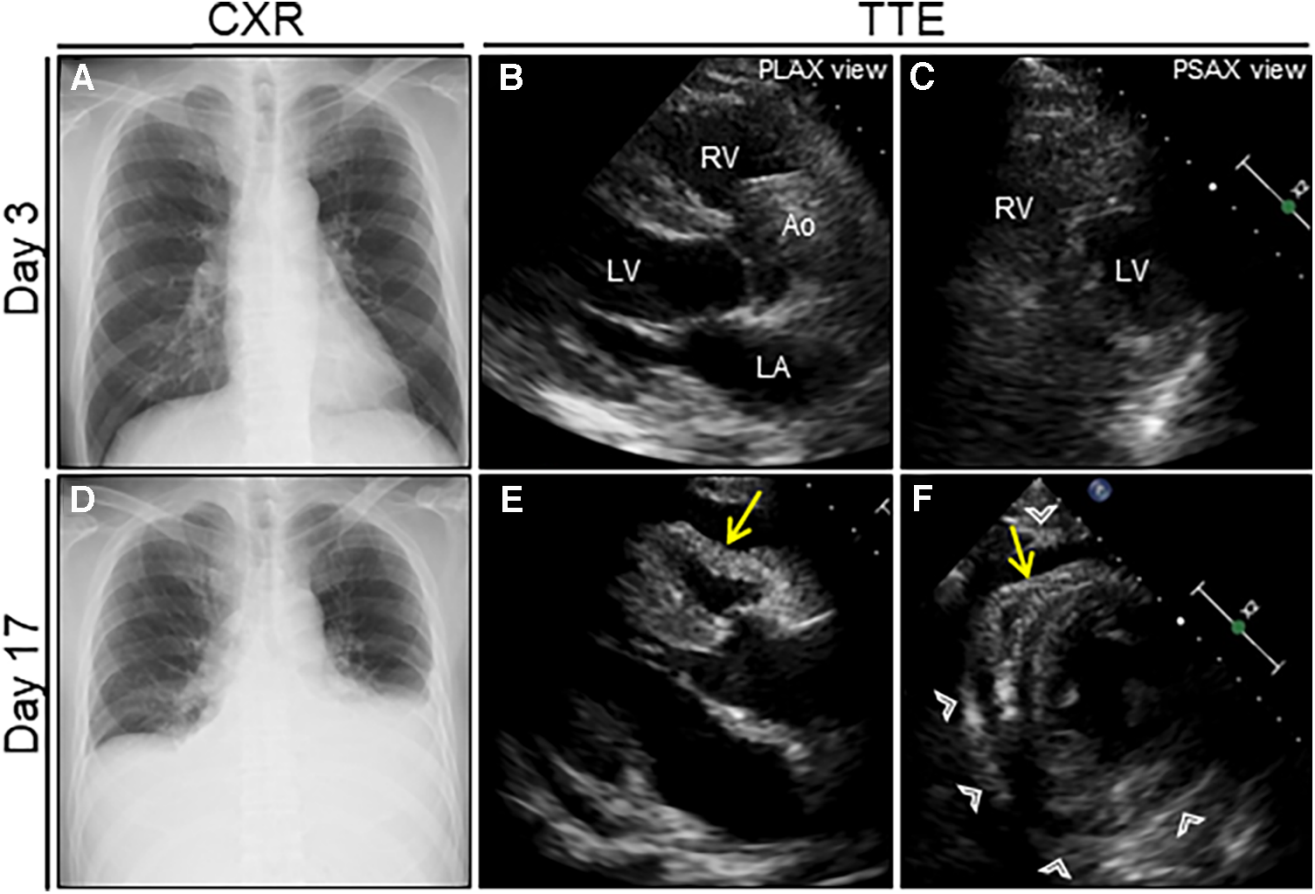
Serial chest radiograph (CXR) and transthoracic echocardiography (TTE) after nosocomial infection of COVID-19. On day 3 after admission (1 day after nosocomial infection of SARS-CoV-2), CXR (A) and TTE (B, C) are unremarkable. On day 17 (15 days after nosocomial infection of SARS-CoV-2), the follow-up CXR reveals cardiac enlargement with bilateral pleural effusions (D), whereas the follow-up TTE reveals a moderate pericardial effusion with pericardial thickening (arrowheads) (E, F). Note the right ventricular collapse during early diastole (arrows). Ao, aorta; COVID-19, coronavirus disease; LA, left atrium; LV, left ventricle; PLAX, parasternal long-axis; PSAX, parasternal short-axis; RV, right ventricle; SARS-CoV-2, severe acute respiratory syndrome coronavirus 2.
